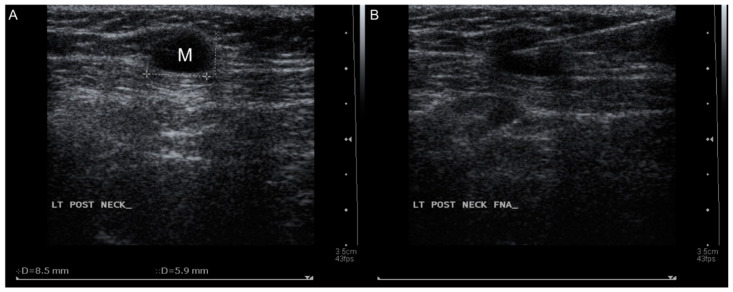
(A) Targeted ultrasound revealed an ovoid hypovascular hypoechoic mass with a short axis of about 0.6 cm and preserved. (B) Fine needle aspiration cytology was performed using a 21-gauge needle.
